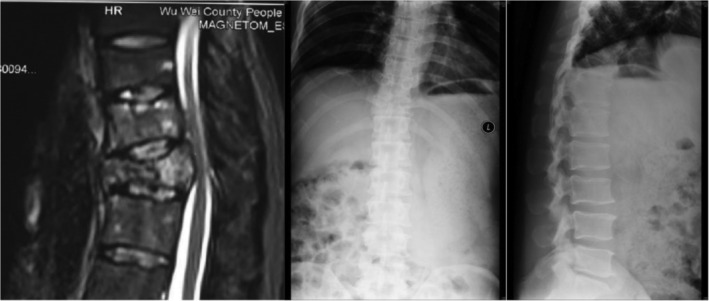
Preoperative MRI and X‐ray scan.
